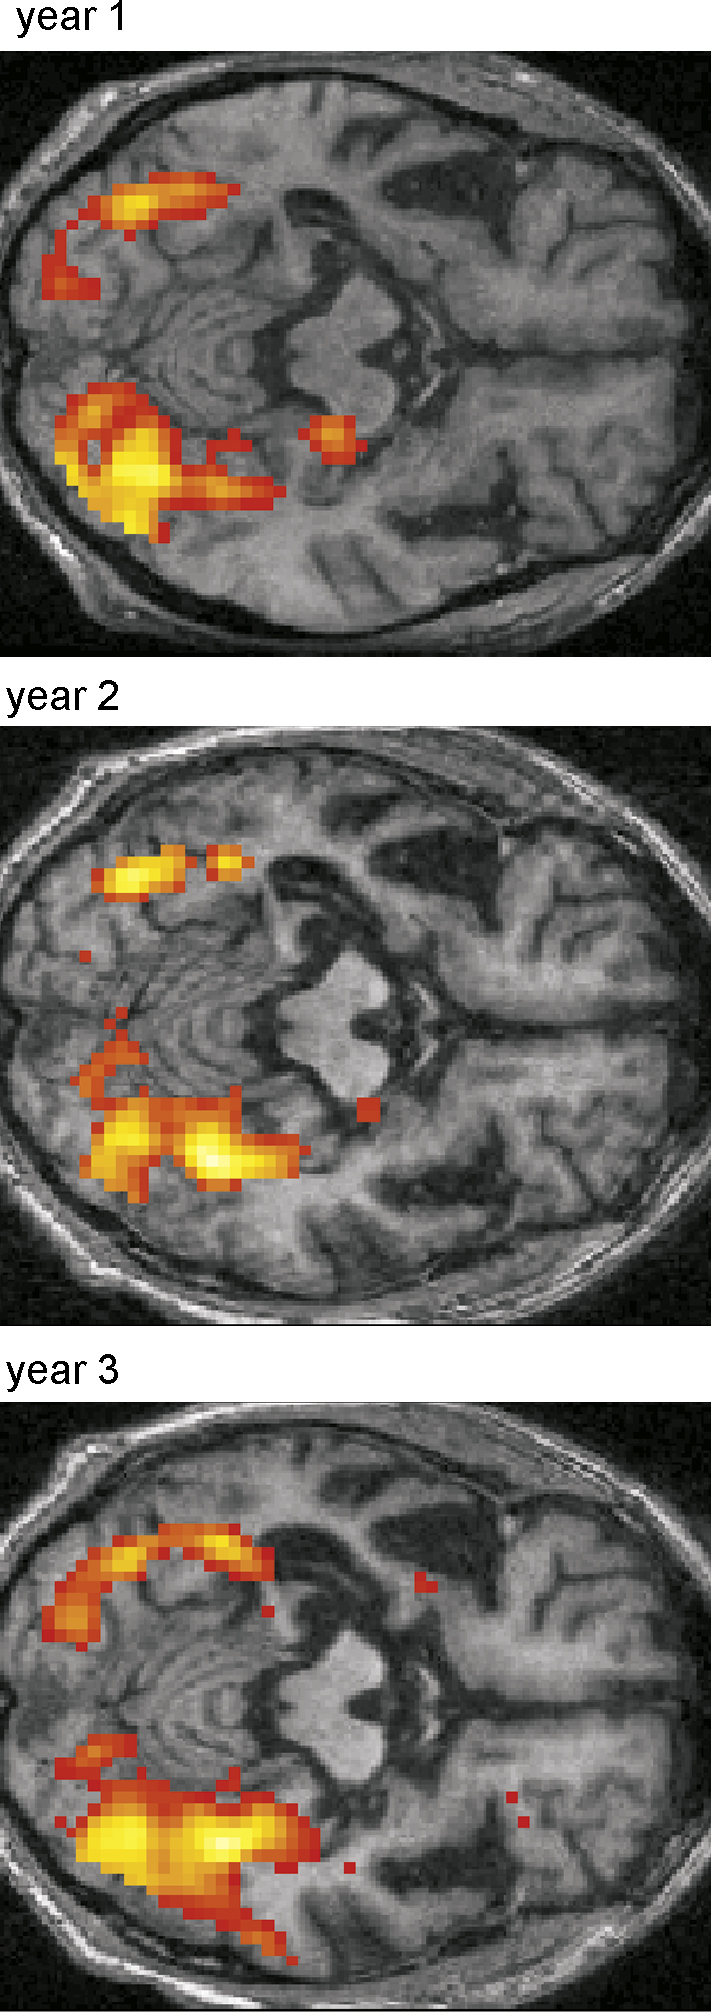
The brain areas associated with processing foil photographs. Brain areas more active during processing of foil photographs compared with the control task are shown for each year. Functional images are shown on an axial section from the patient's structural MRI scan contemporaneous with the functional images for that year. Activations are shown at a threshold of *p* < 0.005 uncorrected. For year 1, brain areas activated were: right hippocampus (36, −33, −12; *Z* = 3.35); left posterior parahippocampal gyrus/fusiform cortex (−36, −42, −27; *Z* = 5.16); left lateral occipital cortex/posterior fusiform cortex (−42, −54, −18; *Z* = 5.65); right lateral occipital cortex/posterior fusiform cortex (36, −54, −21; *Z* > 8); right, superior part of middle occipital gyrus (33, −84, 9; *Z* > 8); right middle occipital gyrus (45, −81, −9; *Z* > 8); left inferior occipital gyrus (−36, −78, −21; *Z* > 8). Year 2: right hippocampus/parahippocampal gyrus (35, −36, −16; *Z* = 4.0); left posterior parahippocampal gyrus/fusiform cortex (−36, −42, −27; *Z* = 5.71); right fusiform cortex (36, −54, −21; *Z* > 8); left lateral occipital cortex/posterior fusiform cortex (−42, −48, −21; *Z* = 5.61); right lateral occipital cortex/posterior fusiform cortex (36, −54, −21; *Z* > 8); right, superior part of middle occipital gyrus (33, −84, 6; *Z* = 5.83); right middle occipital gyrus (45, −78, −9; *Z* > 8); left inferior occipital gyrus (−36, −75, −21; *Z* = 7.62). Year 3: left posterior parahippocampal gyrus/fusiform cortex (−36, −42, −27; *Z* = 7.64); right posterior parahippocampal gyrus/fusiform cortex (29, −35, −26; *Z* = 5.0); right fusiform cortex (33, −48, −18; *Z* = 7.1); left lateral occipital cortex/posterior fusiform cortex (−42, −51, −18; *Z* = 6.36); right lateral occipital cortex/posterior fusiform cortex (36, −51, −18; *Z* > 8); right, superior part of middle occipital gyrus (30, −84, 6; *Z* = 7.76); right middle occipital gyrus (45, −81, −9; *Z* > 8); left inferior occipital gyrus (−36, −78, −21; *Z* = 6.24). Cross-year comparisons revealed only one significant result: foil-control task year 1 > foil-control task year 3, right hippocampus (21, −27, −15; *Z* = 4.80).
